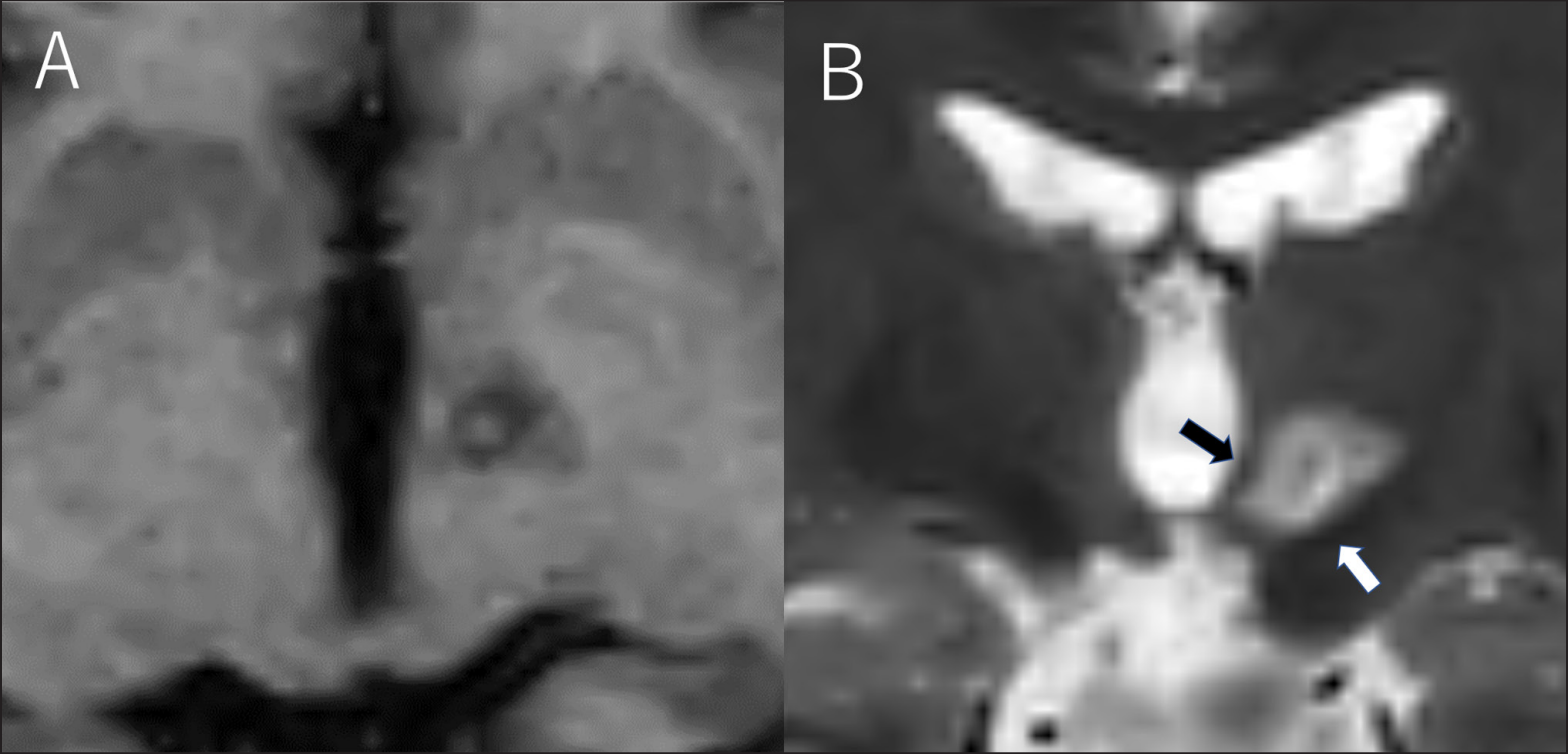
Postoperative MRI. T1-axial (A) and T2-coronal (B) MRI showing a lesion on the left pallidothalamic tract. Black arrow showing the mammillothalamic tract and white arrow showing the subthalamic nucleus.
